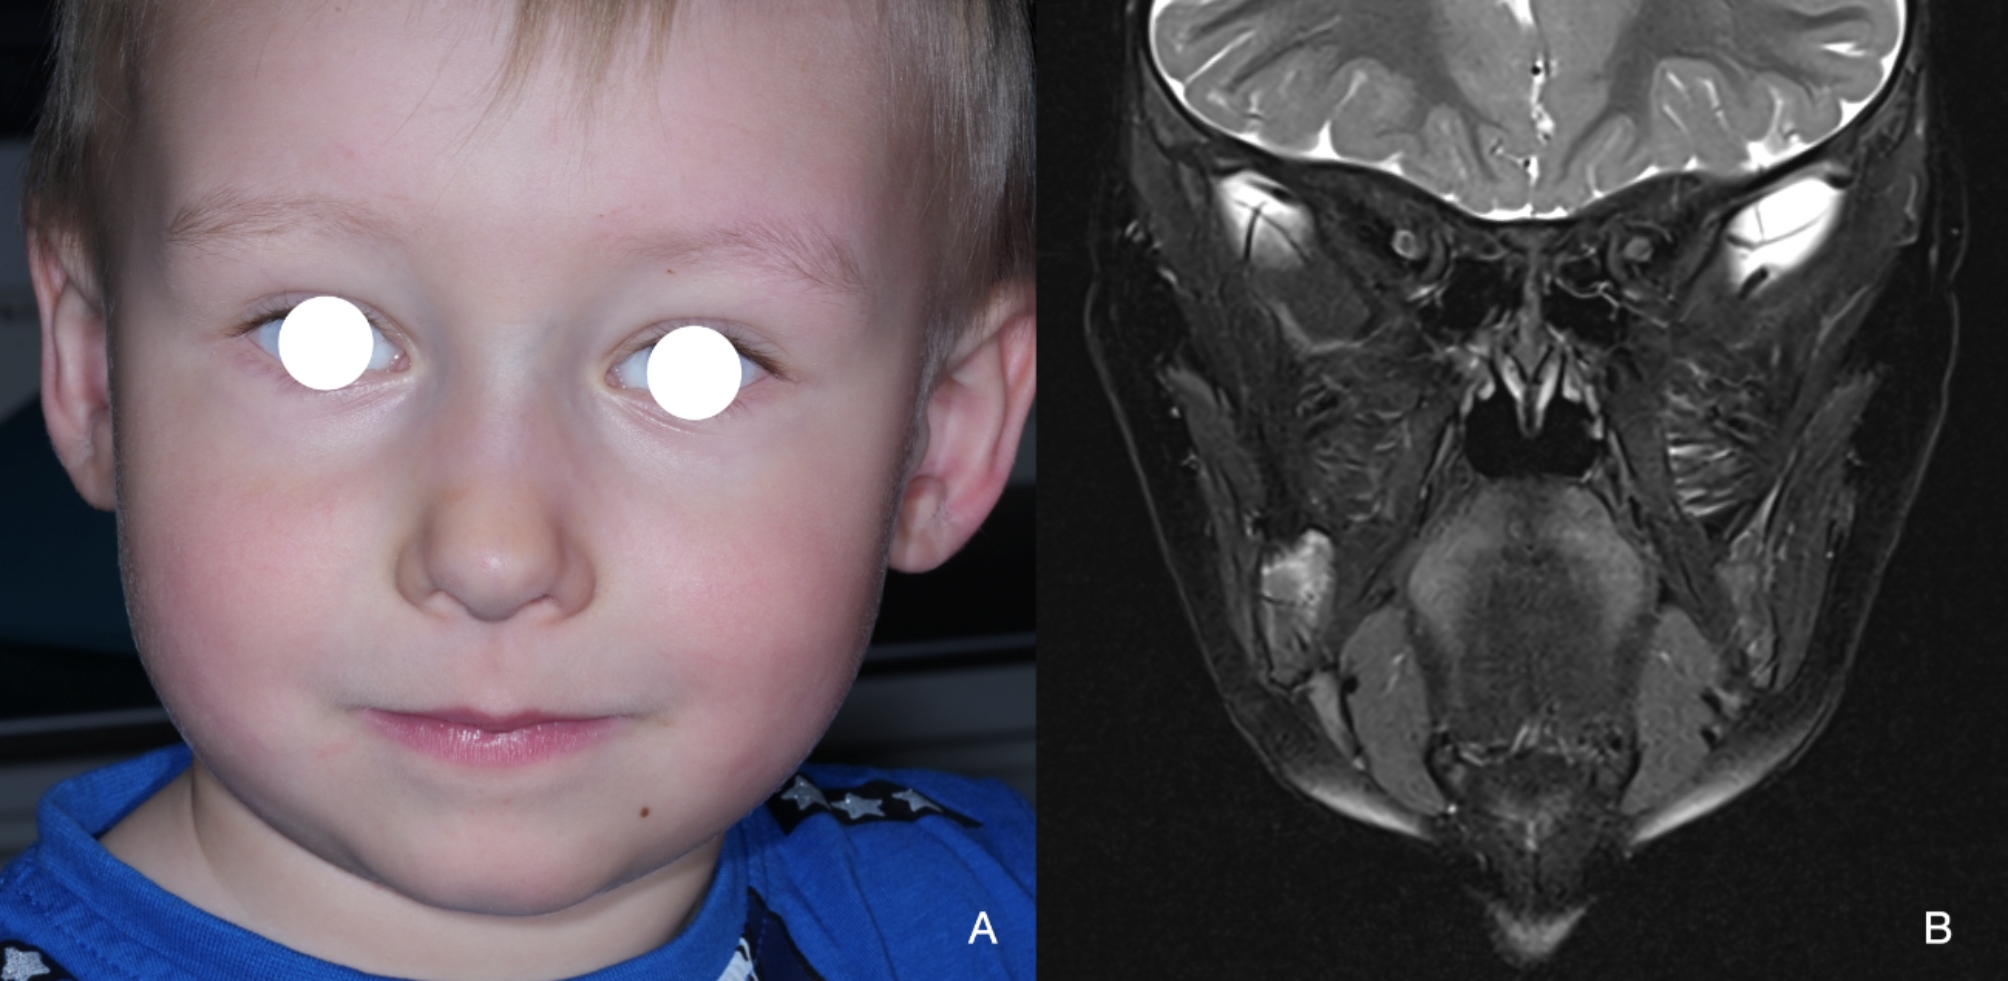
Post-operative follow-up at six months. A, clinical aspect with resolution of facial asymmetry at cheek level. B, MRI showing no recurrence or tumor persistence but signs of bone remodeling
